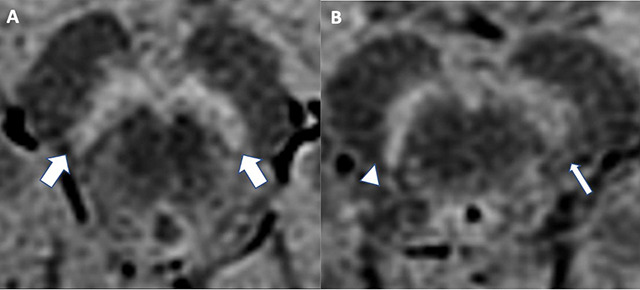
Neuromelanin. Assessment of the substantia nigra using 3T high resolution T1-weighted MR imaging in the midbrain structures in 2 different cases. The control subject (A) shows normal nigral hyperintensity present bilaterally (thick arrow) and the PD patient (B) demonstrates right loss of dorsolateral nigral hyperintensity (arrowhead) and left dorsolateral nigral hyperintensity reduced (thin arrow).
